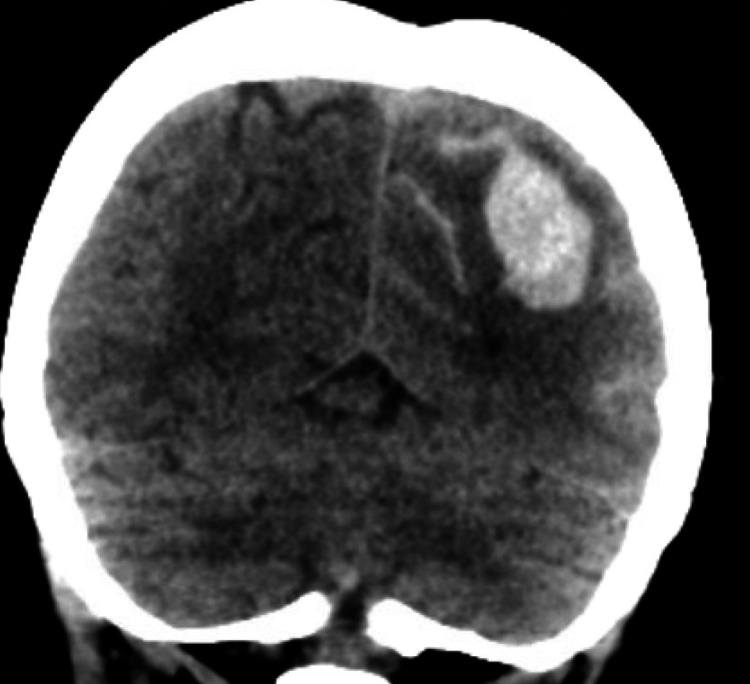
Computed tomography of the head demonstrating diffuse left-sided subarachnoid hemorrhage extending to the left ambient cistern, along with a 3.2 × 1.8 cm intraparenchymal hemorrhage at the left posterosuperior parietal lobe without midline shift.
